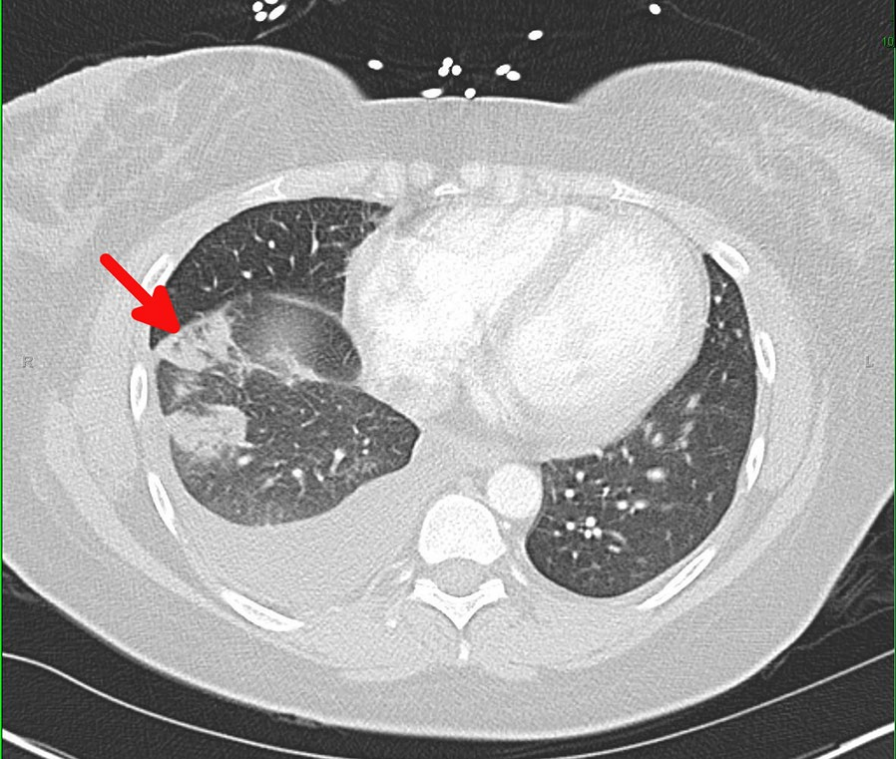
CT chest without contrast (*transverse view*) demonstrating a *right* lower lobe wedge-shaped pulmonary infarction (*red arrow*)
